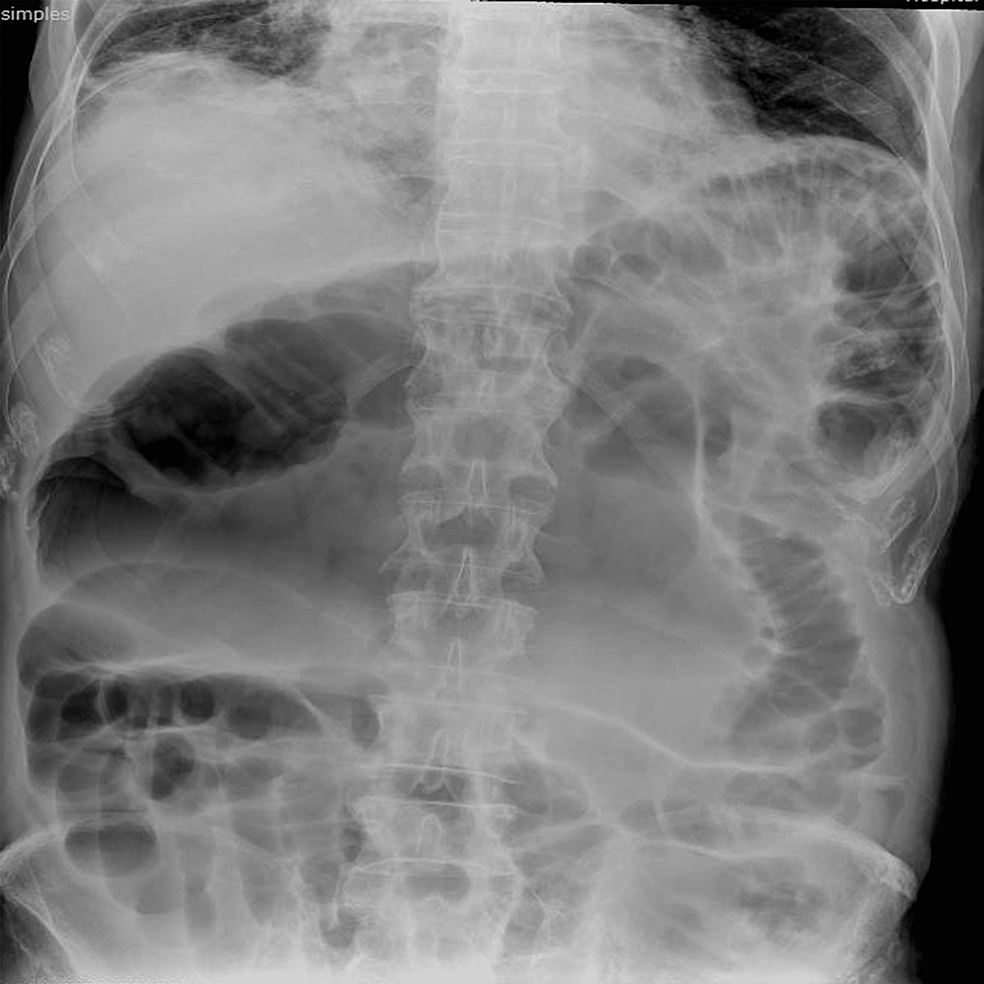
Abdominal X-ray revealing dilated large bowel loops
